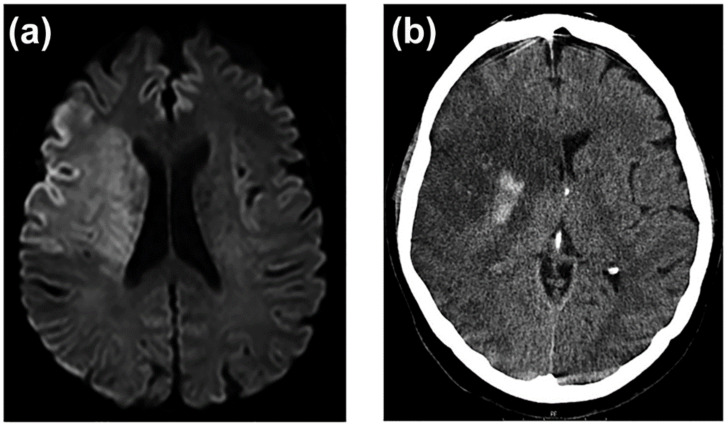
A 61-year-old woman with cardioembolic stroke in the territory of the middle cerebral artery. (a) In the first DWI-MRI (left) that corresponds to a cytotoxic oedema. (b) The same patient at 48 h (right). CT shows intense vasogenic oedema and hemorrhagic transformation of the infarct.
